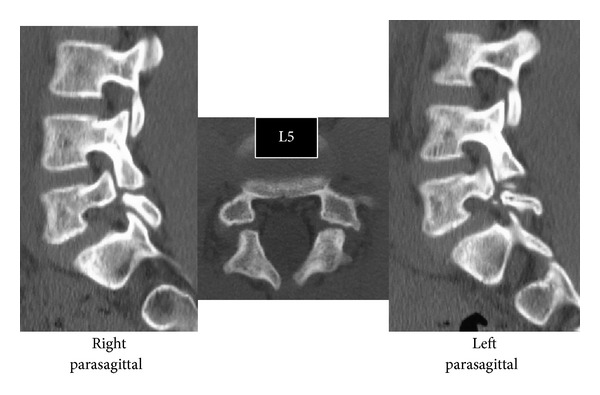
CT of Case 1. L5 spondylolysis is considered to be at the terminal stage (i.e., pseudoarthrosis).
